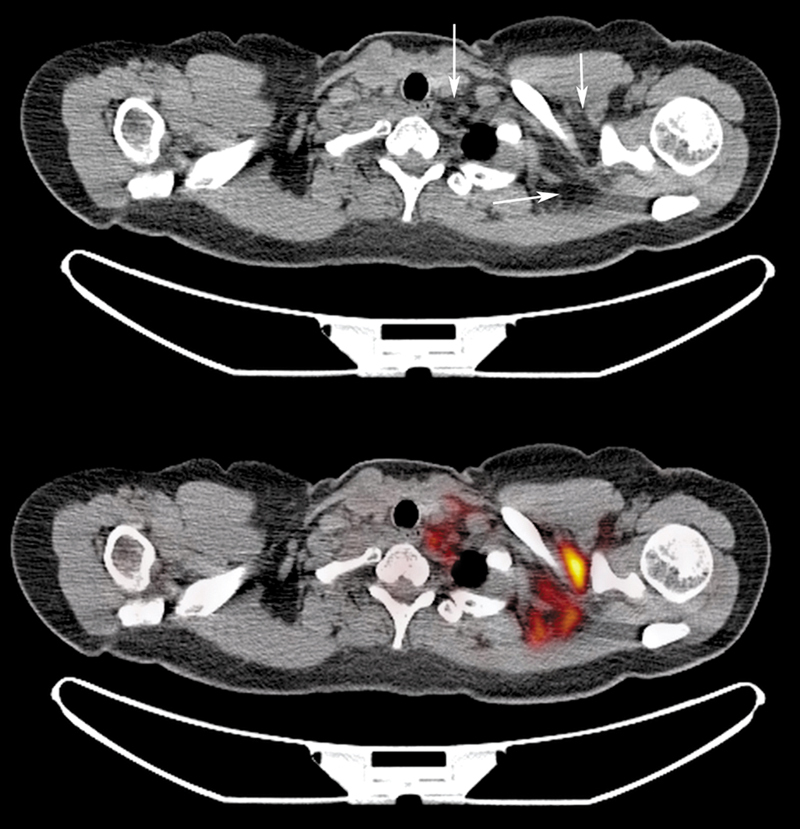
Axial slices at the level of left clavicle demonstrating low attenuating tissue without evidence of lymphonodal disease in the left supraclavicular, posterior cervical and upper left mediastinal regions (white arrows, upper row) in regions with focal FDG uptake (lower row).
